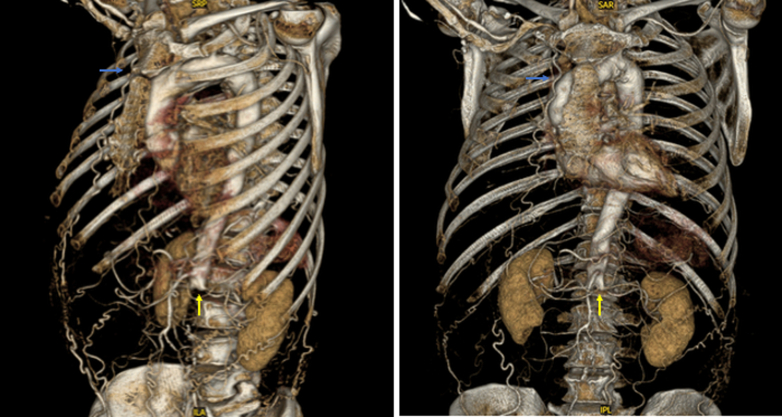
3-Dimensional Reconstruction of Computed Tomography Aortogram With Arterial Contrast Images demonstrate total occlusion of the infrarenal aorta and bilateral iliac arteries (yellow arrows). Contrast opacification is absent distal to the occlusion point just below the renal artery origins. The lumbar and intercostal arteries are faintly visualized, consistent with collateralization. The engorged right internal mammary artery is well visualized (blue arrows), as are its collateralized connections with vessels of the anterior abdominal wall (Winslow pathway).
